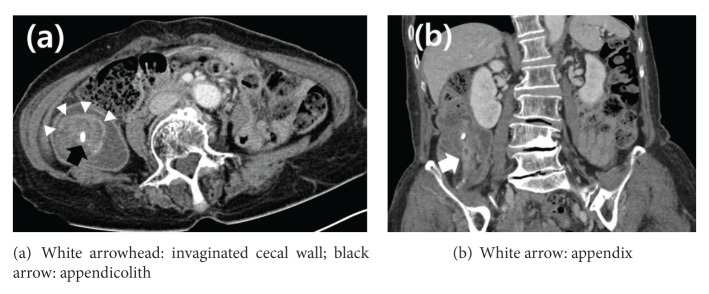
Abdominal computed tomography. Transverse (a) and coronal (b) views revealed invagination of the caecum and the appendix into the lumen of the ascending colon, as well as mural thickening and enhancement of the dilated appendix (9.7 mm) with a few appendicoliths.
